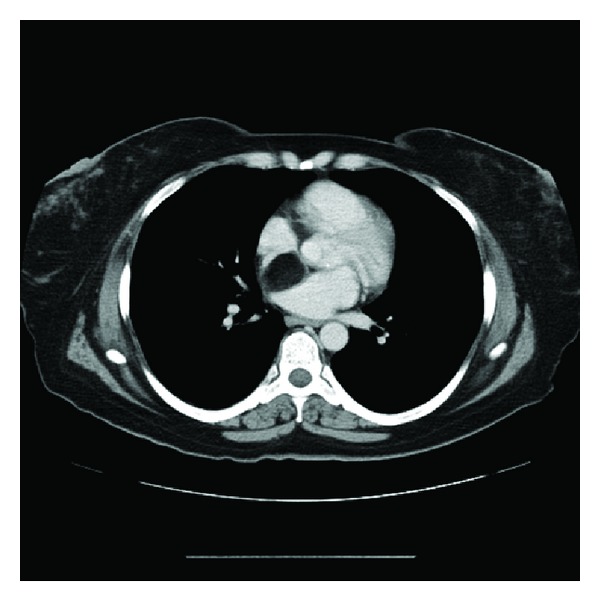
Axial CT image with intravenous contrast, showing the interatrial septal lipoma (encapsulated).
